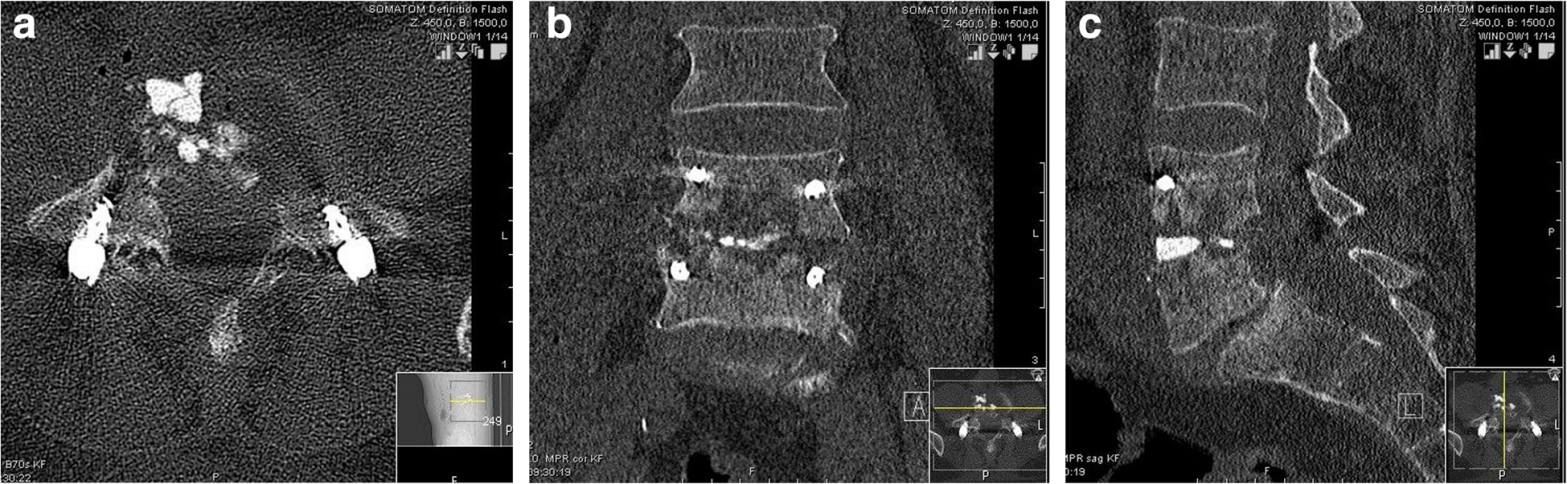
a, b and c: Postoperative computed tomography scan on 9 Jan 2015 (axial (*left*), coronary (*middle*) and sagittal (*right*) reconstructions): correct position of transpedicular screw-rod instrumentation. Intervertebral space was filled with the antibiotic-eluting bone graft substitute, which contains a radio contrast agent (Iohexol) with good visibility (especially on sagittal reconstruction)
